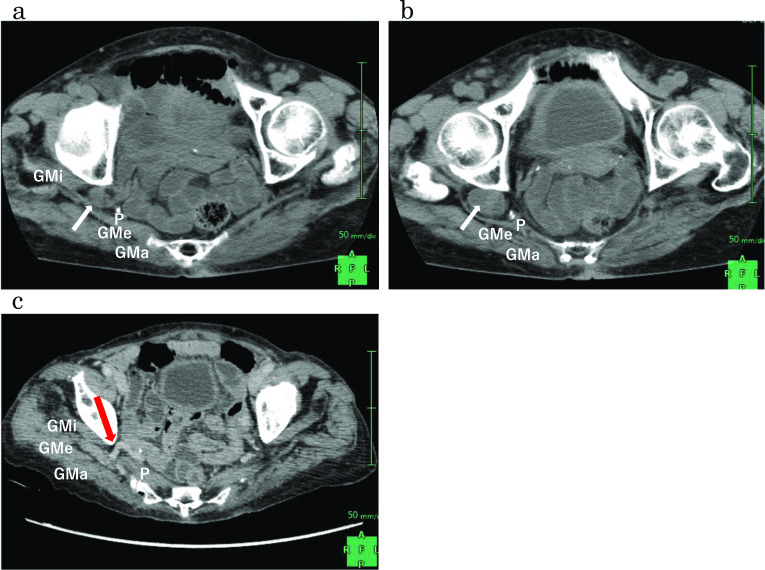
CT findings. a, b The small intestine herniated into the right greater sciatic foramen above the piriformis muscle (arrow). *P* piriformis muscle, *GMa* gluteus maximus muscle, *GMe* gluteus medius muscle, *GMi* gluteus minimus muscle. c A dilated superior gluteal vein was manifested when small bowel herniation was not observed in the right suprapiriform foramen (red arrow)
